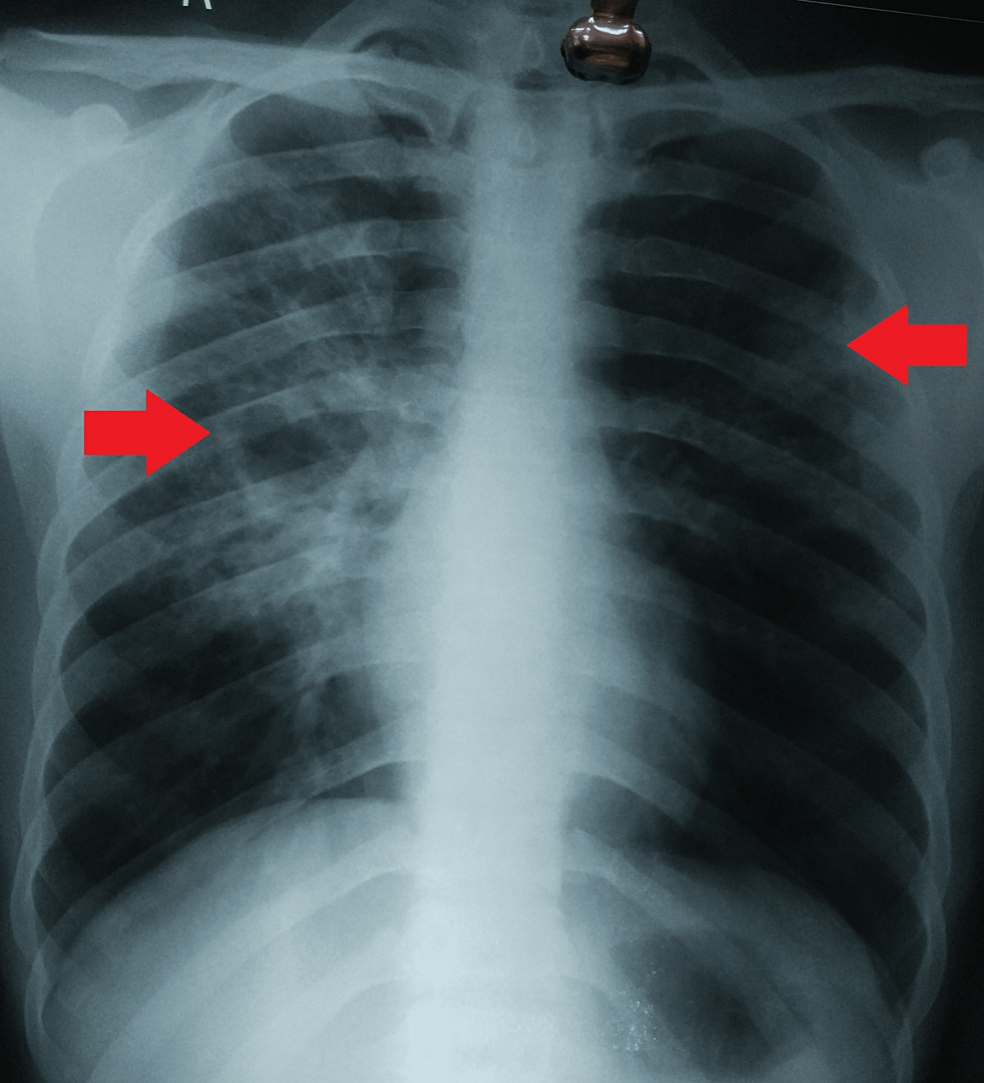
Chest radiograph posteroanterior view suggestive of bilateral lung involvement with patchy opacities and right-sided cavity
